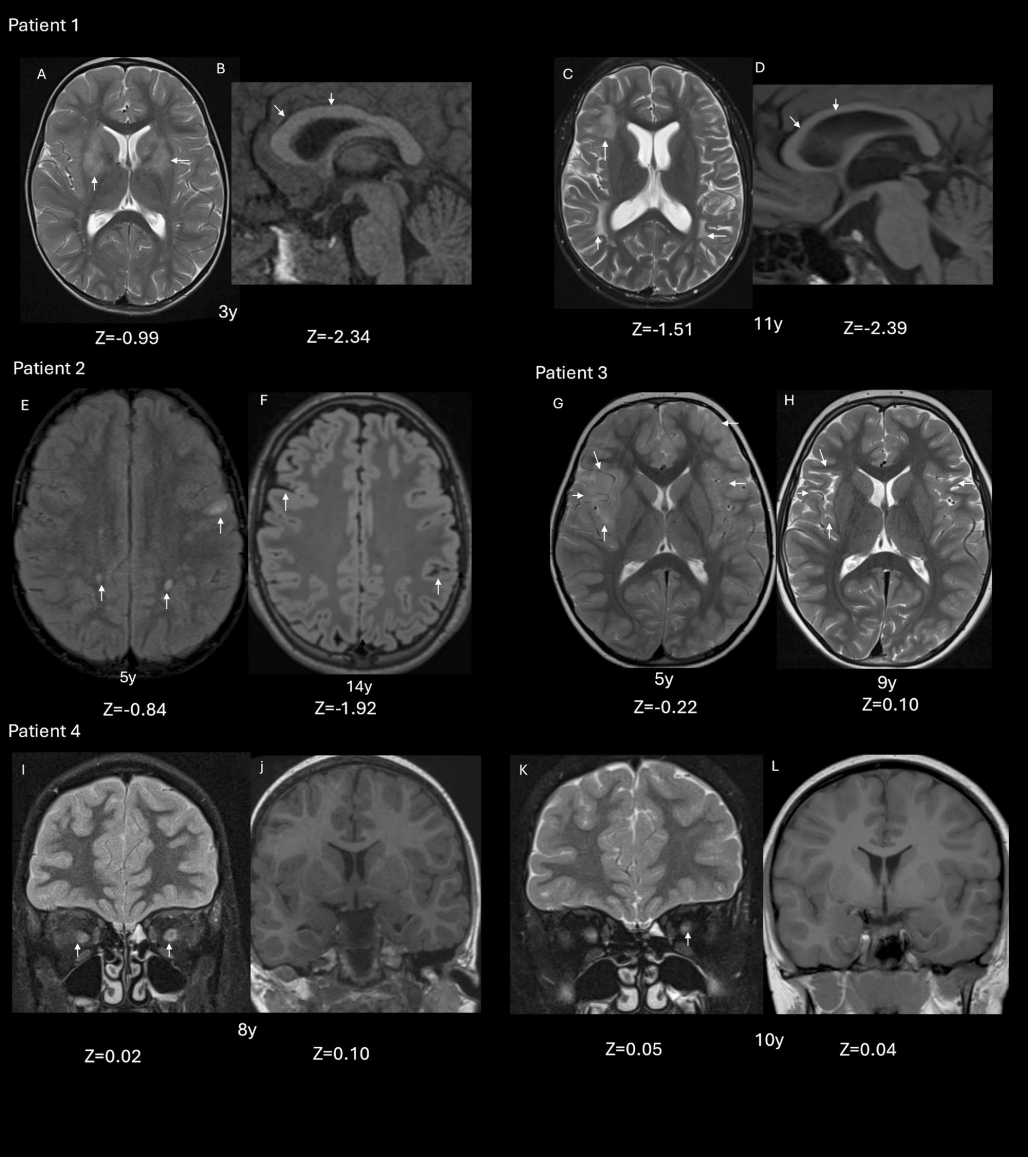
Iconographic representation of paediatric MOGAD patients. Patient 1: at the onset of disease, 3 years of age: axial T2 weighted image at the level of the basal ganglia (A) shows hyperintense lesions involving the caudate and putamina bilaterally (arrows, A). The overall brain volume is preserved with no sulcal or ventricular dilatation. Note the normal appearances of the corpus callosum (arrows, B). Follow‐up imaging, 11 years of age: Axial T2 weighted image at the level of the basal ganglia shows that the caudate and putaminal lesions have now resolved. There are, however, new white matter lesions in the frontal and temporoparietal white matter (arrows, C). Note diffuse volume loss evidenced by the prominence of the ventricles and sulcal spaces (C), and white matter volume loss represented by callosal thinning on the sagittal T1 weighted image (D). Patient 2: At onset of disease, 5 years of age: Axial FLAIR sequence shows multiple white matter lesions involving the frontal and parietal white matter (arrows, E). The overall brain volume is preserved with no sulcal dilatation. Follow up imaging, 14 years of age: Axial FLAIR sequence shows resolution of the white matter lesions. There is now an overall decrease in brain volume evidenced by prominent sulcal spaces in (arrows F). Patient 3: At onset of disease, 5 years of age. Axial T2 weighted image shows swelling and hyperintensity involving the bilateral fronto‐insular and opercular cortices (right > left) (arrows, G). On follow up imaging at 9 years of age, there is no whole brain volume loss with resolution of previously seen lesions (arrows, H). Patient 4: Coronal saturated T2 weighted image shows bilateral optic neuritis (arrows, I). Coronal T1 weighted image shows preserved brain volume. Brain volume is preserved (J). Follow up imaging at 10 years of age shows residual signal change within the optic nerves with atrophy (arrow, K). No brain volume loss is evident (L).
